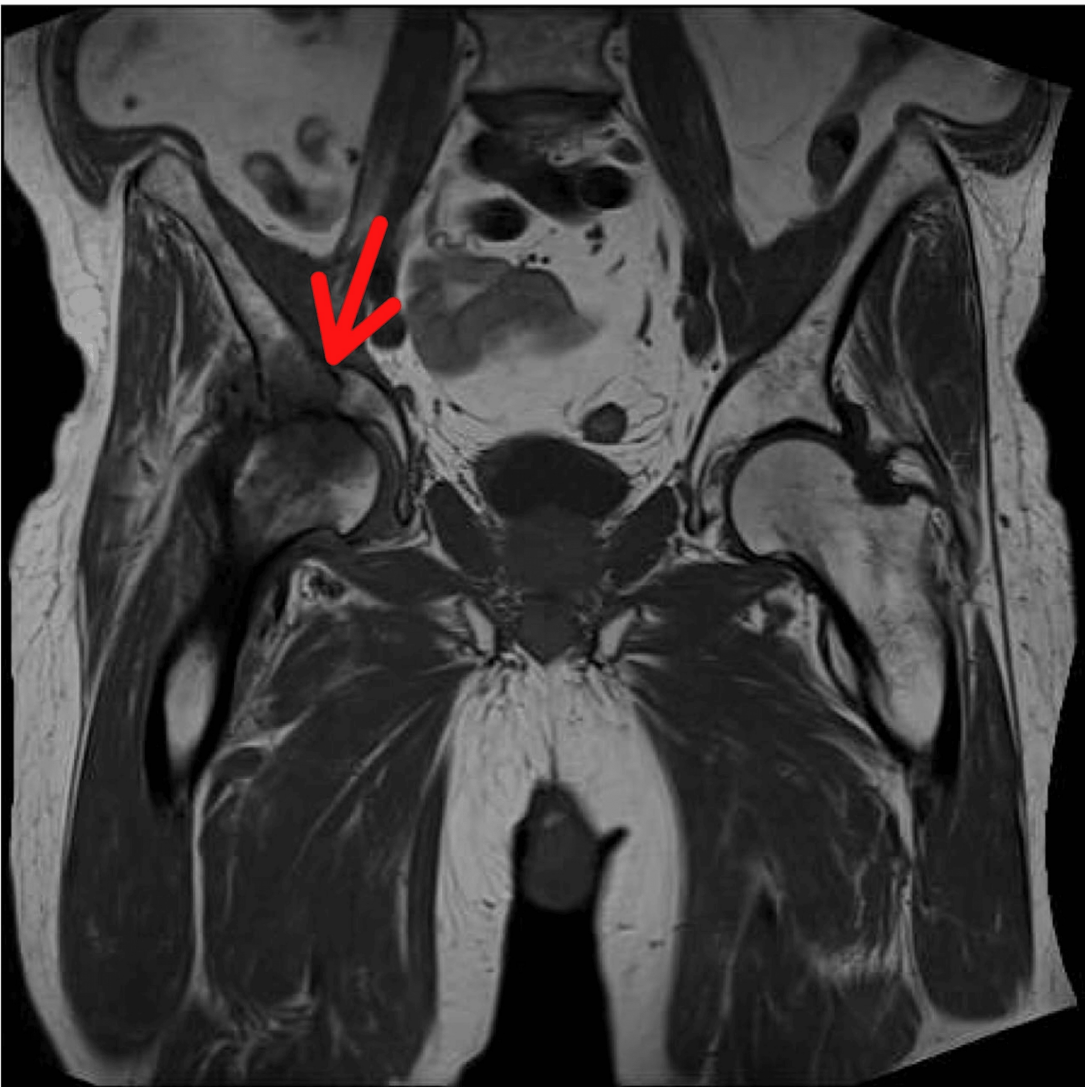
MRI pelvis showing a fracture of the right acetabulum (red arrow) after radiotherapy for prostate cancer. The examination was performed on October 27, 2023, in the radiology department at Bolton NHS Trust, UK.
